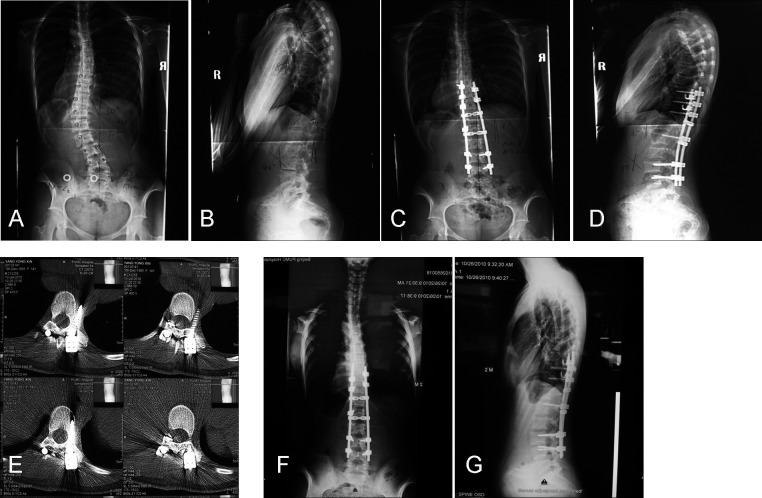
Patient is a 15 year-old female with Marfan syndrome with scoliosis. A and B, Standing preoperative anteroposterior and lateral radiographs. C and D, Standing anteroposterior and lateral radiographs 4 days after operation. E, Magnetic resonance images, showing improper implant location. F and G, Standing anteroposterior and lateral radiographs 4 days after reoperation.
